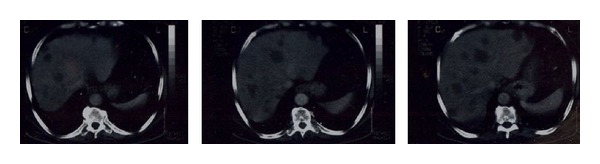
Abdominal CT-scan showing multiple hypodense nodules in both liver lobes.
